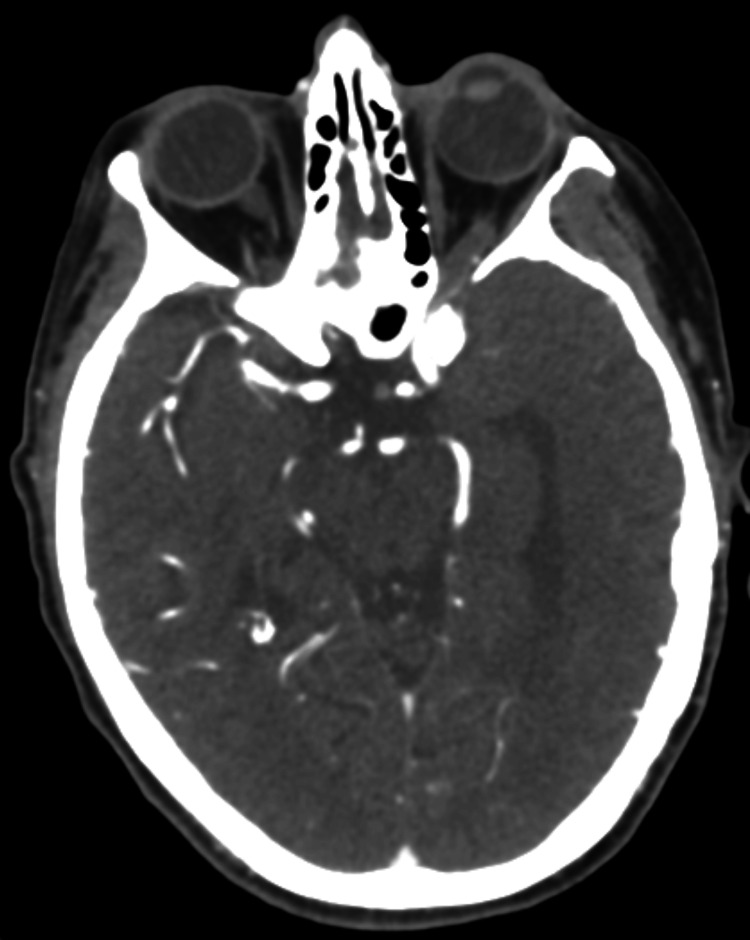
CT Angiogram of the Brain (Axial View) CT angiography of the brain is grossly unremarkable, with no overt abnormal findings. CT: computed tomography
